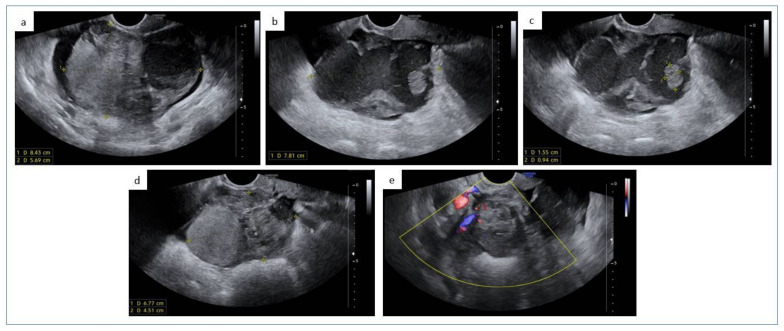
(a–e) In right adnexal site a multilocular solid-cystic mass (88 × 57 × 78 mm) has been observed, with ground grass content, complete/incomplete septa (a,b) and endoluminal papillae (15 × 9 mm, the largest one, (c)). The solid component measured 68 × 45 mm (d) and it was moderately vascularized at Color Doppler (e).
